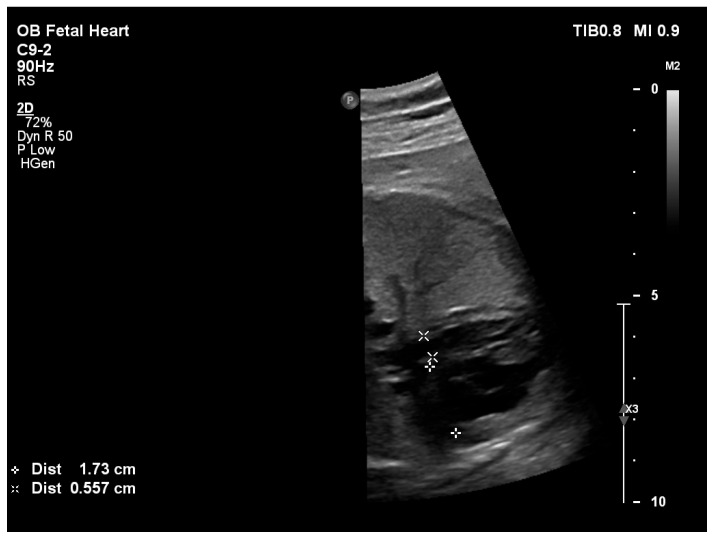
Four-chamber fetal echocardiographic view at 32 weeks’ gestation demonstrating measurement of the mitral valve annulus (0.557 cm) and tricuspid valve annulus (1.73 cm).
